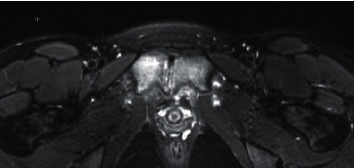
MRI after completion of therapy (about 6 months later).
